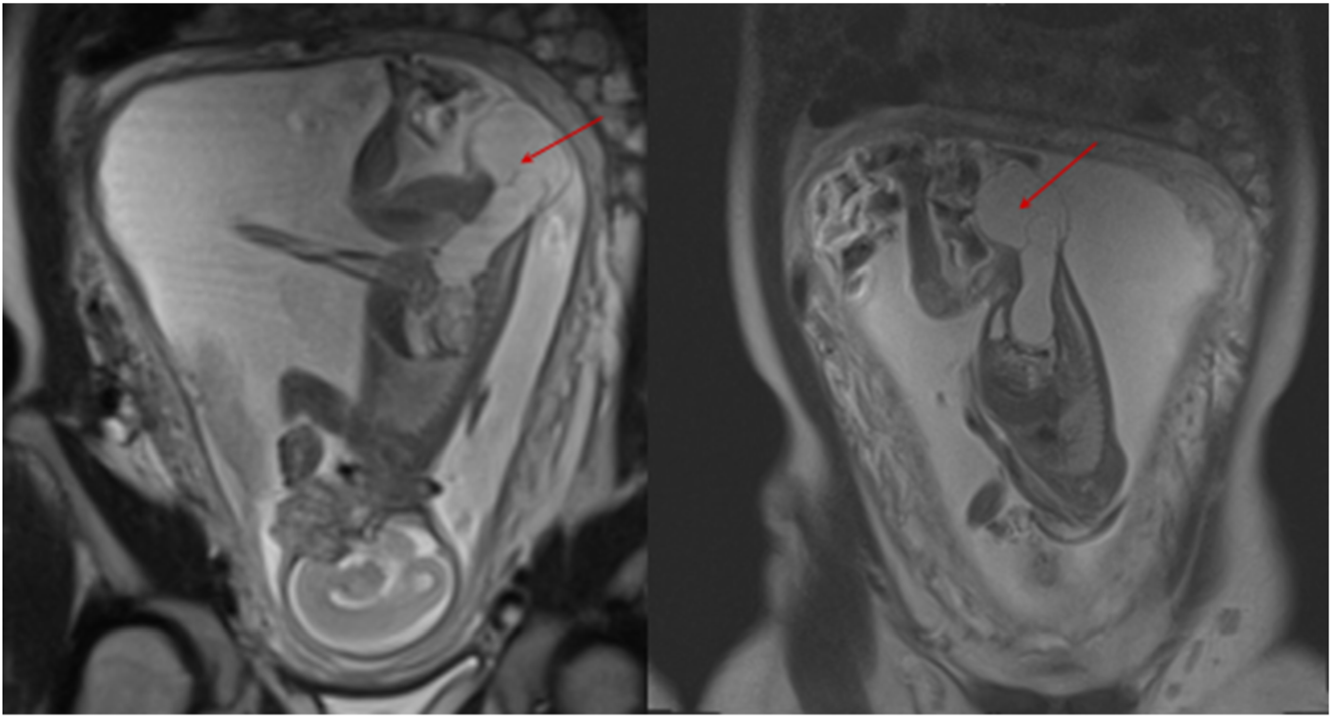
Fetal MRI of a cystic formation in the sacrococcygeal region.
